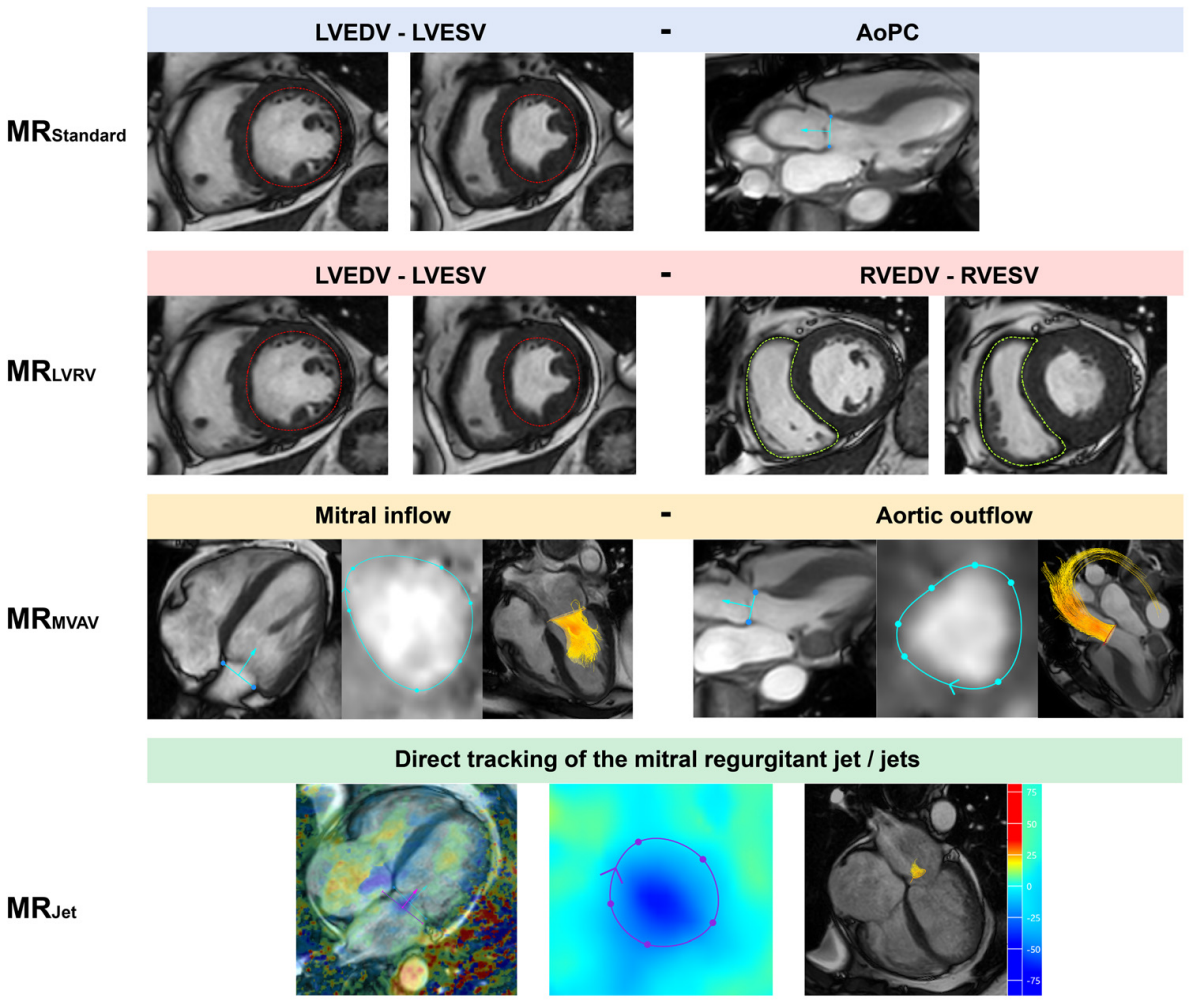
Visual description of the four cardiovascular magnetic resonance imaging mitral regurgitation volume quantification methods investigated within this study. AoPC - aortic phase contrast; LVEDV - left ventricular end diastolic volume; LVESV - left ventricular end systolic volume; RVEDV - right ventricular end diastolic volume; RVESV - right ventricular end systolic volume.
